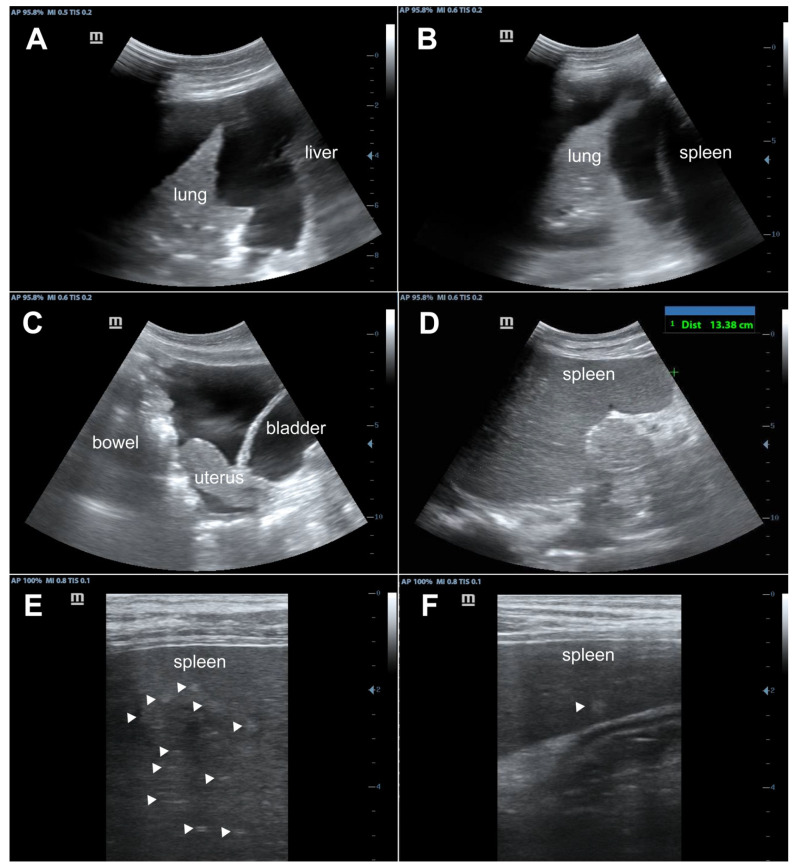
Point-of-care ultrasound performed on Day 2. Findings were consistent with disseminated KS, including right pleural effusion (A); left pleural effusion (B); pelvic free fluid (C); an enlarged spleen (D); and multiple hyperechoic splenic lesions (the arrowheads in E,F).
